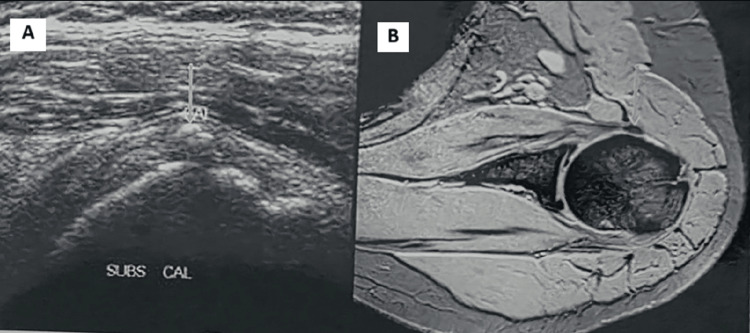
Calcific tendinitis involving the subscapularis tendon: (A) USG showing calcific density foci in the subscapularis tendon near its insertion. (B) Corresponding axial T2FFE MRI image showing blooming in the subscapularis tendon near its insertion T2FFE, T2-weighted fast field echo; USG, ultrasonography; MRI, magnetic resonance imaging
